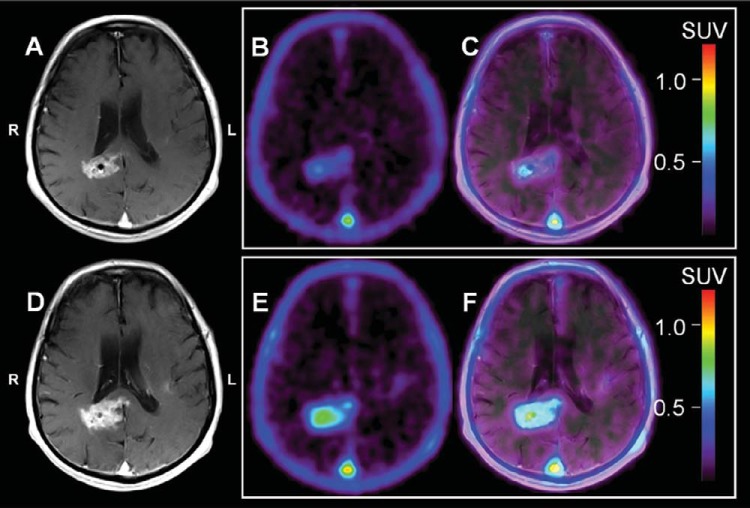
Patient ML-10 #1. Representative baseline (BL; A–C) and early-therapy assessment (ETA; D–F) contrast-enhanced (CE) magnetic resonance imaging (MRI), [^18^F]ML-10 standard uptake value (SUV), and positron emission tomography (PET)/CE-MRI fusion images for a 72-year-old male with a new glioblastoma multiforme (GBM) diagnosis. All images are shown coregistered to the patient's BL CE-MRI. BL CE-MRI (A) shows enhancement in the region of the right periventricular white matter/splenium of corpus callosum and left insular region. ETA CE-MRI (D) shows an increase in size and contrast enhancement consistent with progression. On PET, BL [^18^F]ML-10 SUV image (B) shows mild therapy-naive uptake (BL SUV_max_ = 0.54) located at the tumor site on PET/CE-MRI (C). The ETA [^18^F]ML-10 SUV image (E) shows increased [^18^F]ML-10 uptake (ETA SUV_max_ = 0.84) at the GBM site (F) consistent with increased apoptosis compared with BL.
